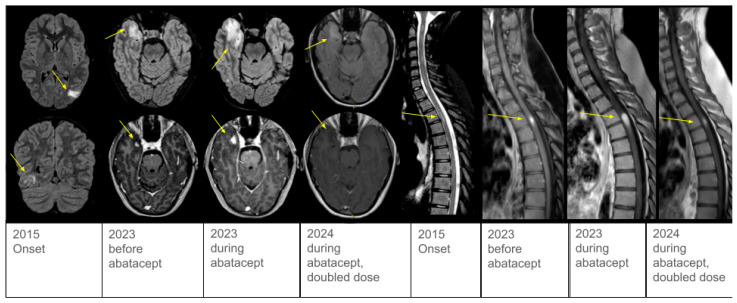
MRI evolution. On the left side: axial FLAIR T2-weighted sequences and post-gadolinium axial 3D IR T1-weighted sequence performed at onset, before abatacept initiation, during standard-dose abatacept therapy, and after dose escalation. On the right side: sagittal cervico-thoracic post-gadolinium 2D T1-weighted images performed at onset, before targeted therapy, during standard-dose abatacept therapy, and after dose escalation. After abatacept initiation, both the right temporal lesion and the spinal lesion worsened, with increased contrast enhancement and perilesional vasogenic edema. All the lesions improved after doubling the abatacept dose.
